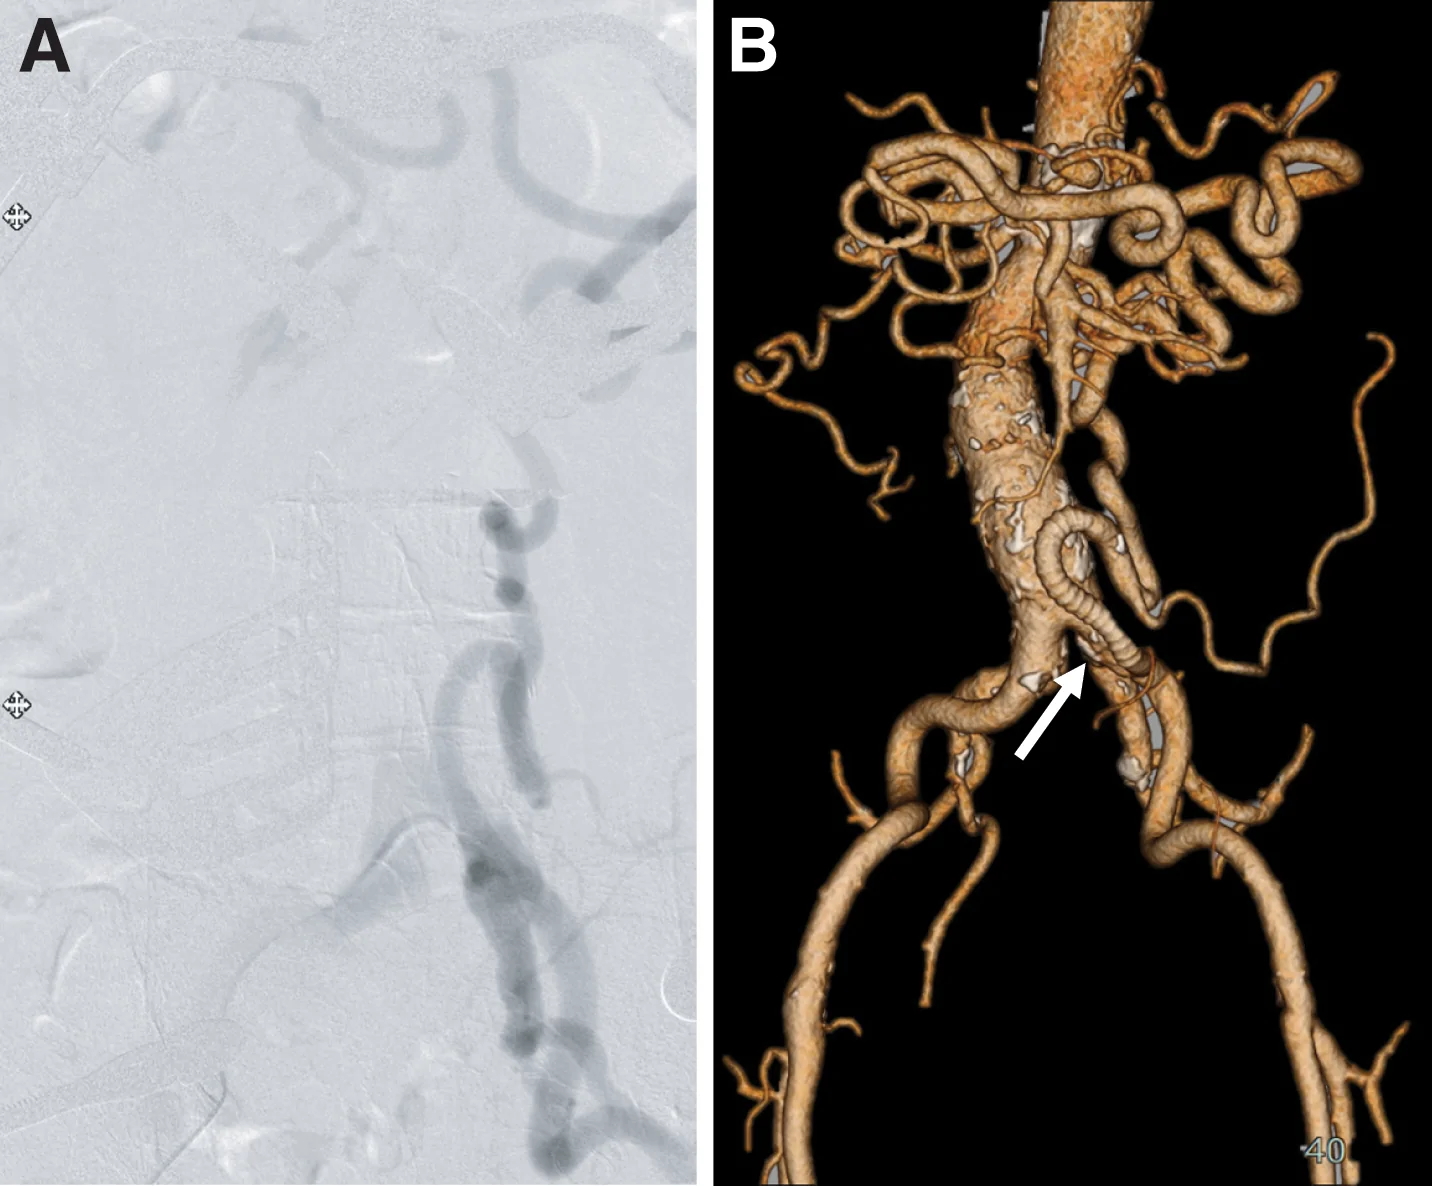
(A) DSA confirms bypass graft patency and adequate perfusion of the CA and SMA territories through the arc of Riolan. (B) Postoperative CT demonstrates patency of the left EIA-to-IMA bypass graft and perfusion of the mesenteric circulation through the IMA branches and dilated marginal arteries (arrow). CA, celiac artery; EIA, external iliac artery; IMA, inferior mesenteric artery; SMA, superior mesenteric artery
